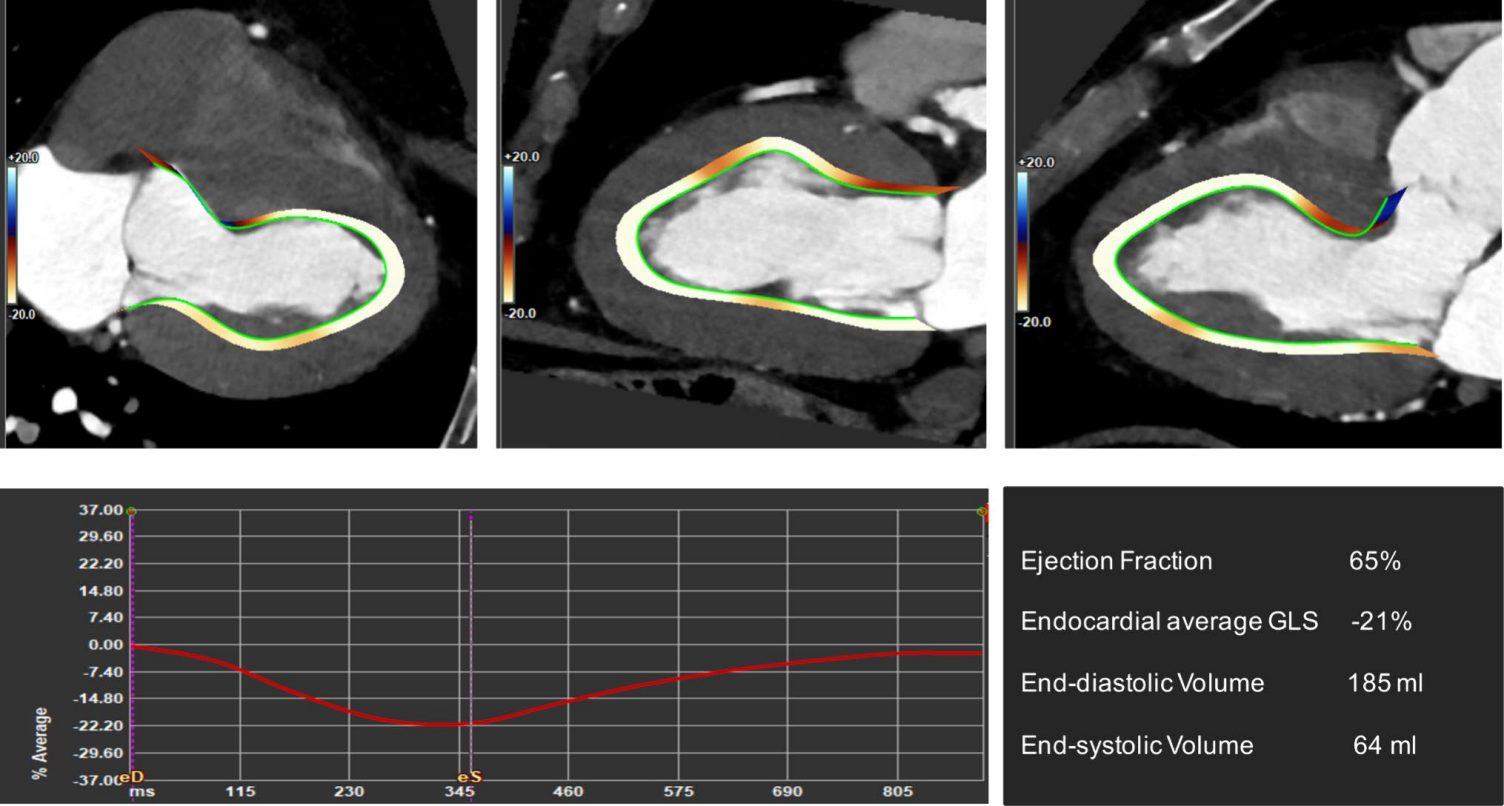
4D CT derived Global Longitudinal Strain (GLS). 67-year-old-male patient with mild aortic stenosis. Positive and negative endocardial end-systolic GLS values are marked in blu and red in the long-axis view (top line). The average GLS-time curve is presented (bottom left) with the prinicpal functional and volumetric parameters derived from the analysis (bottom right) showing normal GLS (-21%) and a normal ejection fraction (65%)
